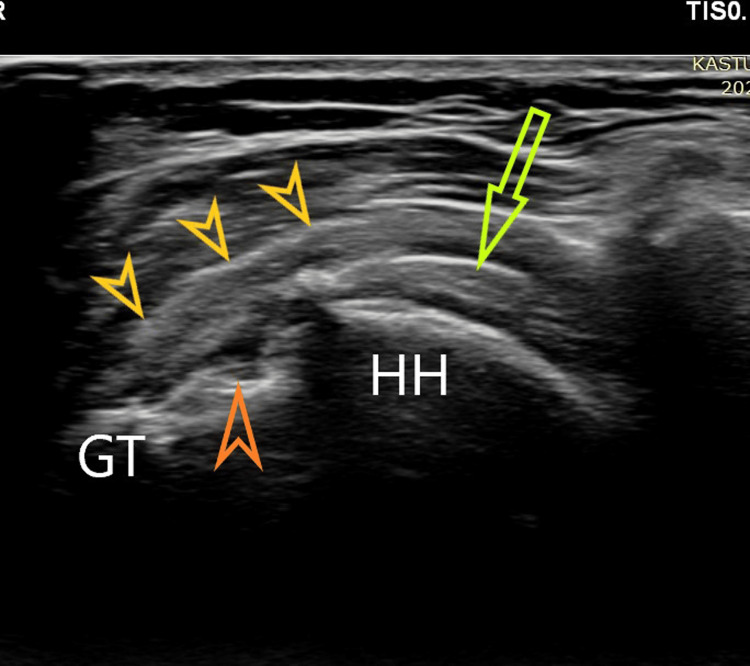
Postoperative ultrasonographic image of a repaired rotator cuff. The yellow arrow indicates the repaired cuff on greater tuberosity, the orange arrow indicates the tip of the anchor inserted on the tuberosity, and the green arrow indicates sutures inside the rotator cuff substance. GT, greater tuberosity; HH, Humeral head
